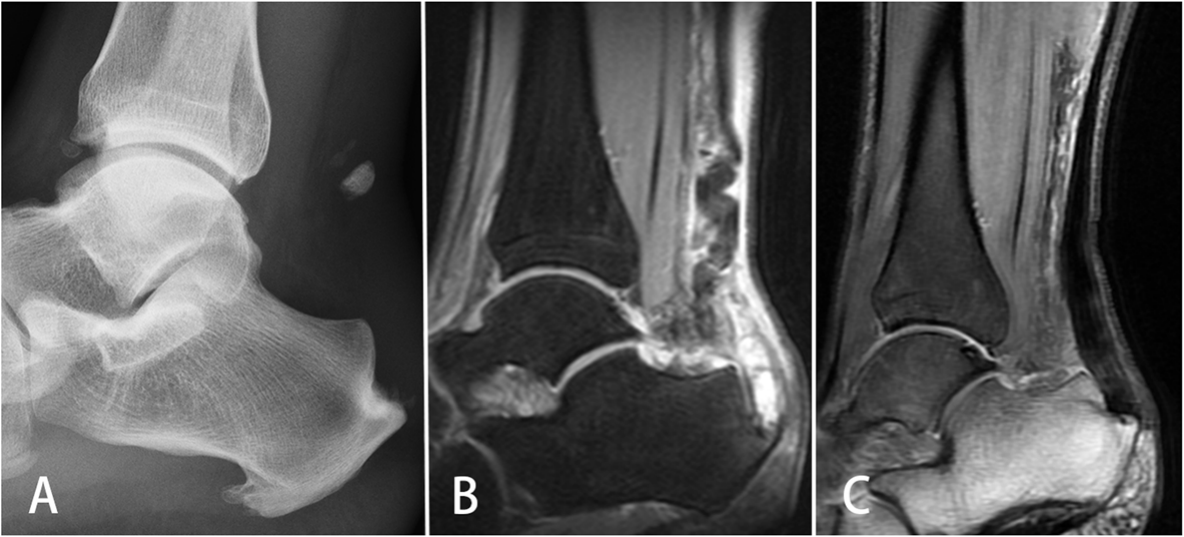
Preoperative and postoperative radiograph and MRI image of a patient (case 1). a Preoperative lateral ankle radiograph of a 34-year-old man who sustained a distal Achilles tendon rupture on his right lower limb 2 days before. An avulsed bone fragment was present with a 5.1-cm shift distance. Calcific spur formation was present at the tendon insertional site. b Preoperative T2-weighted MRI of the ankle demonstrated Achilles tendon rupture and retraction. c Six months after operation, T2-weighted MRI of the ankle demonstrated that Achilles tendon was attached to the calcaneus and the distal part of the Achilles tendon was enlarged
